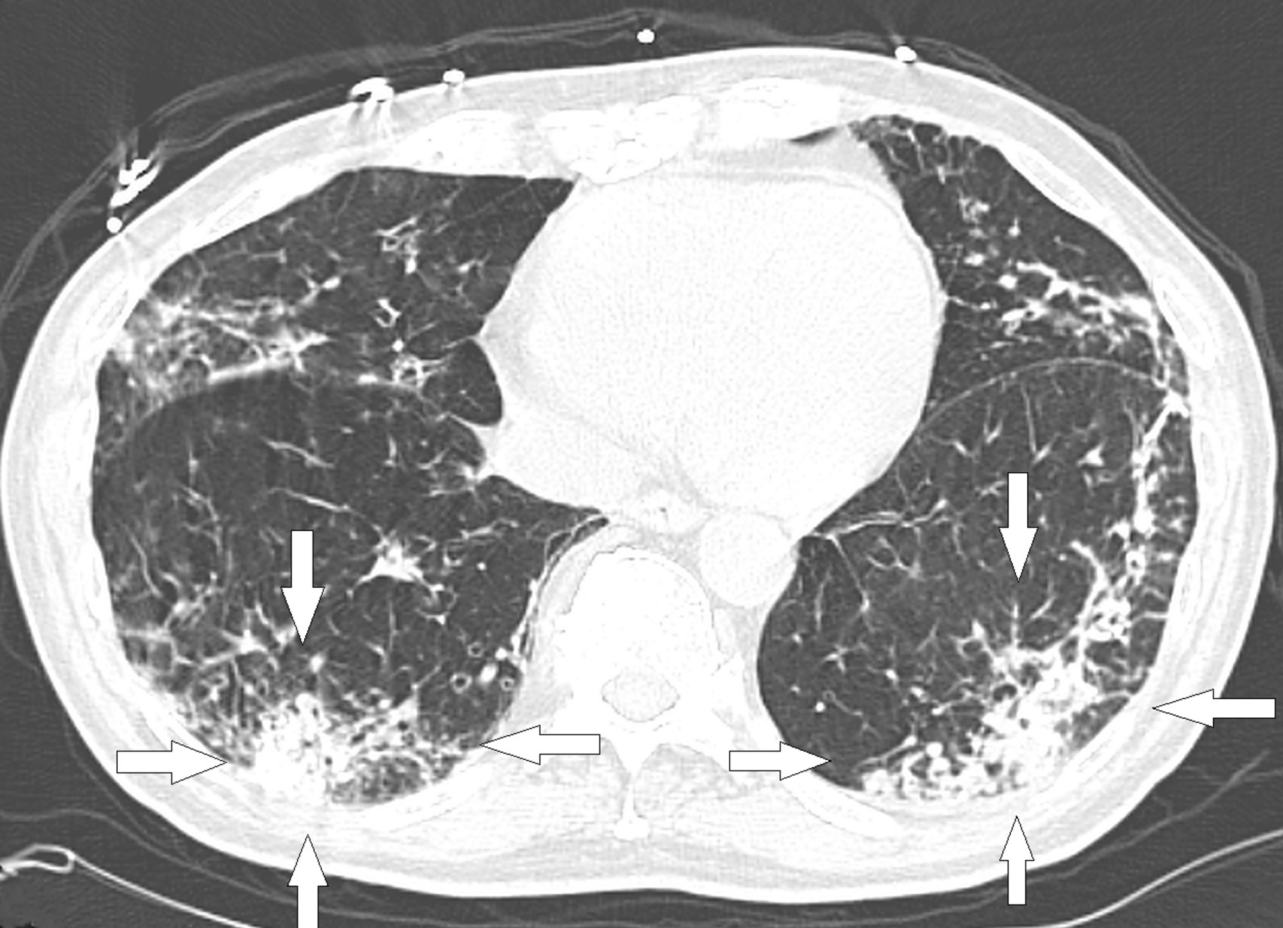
Computed tomography scan showing bilateral lower lobe consolidation
